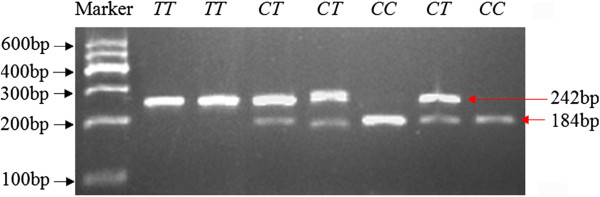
The electrophoresis patterns obtained after digestion with *Sac*I endonuclease at g.2540*C*>*T *locus.*Note*: Fragments including 58 bp of CC and CT genotypes were invisible.
